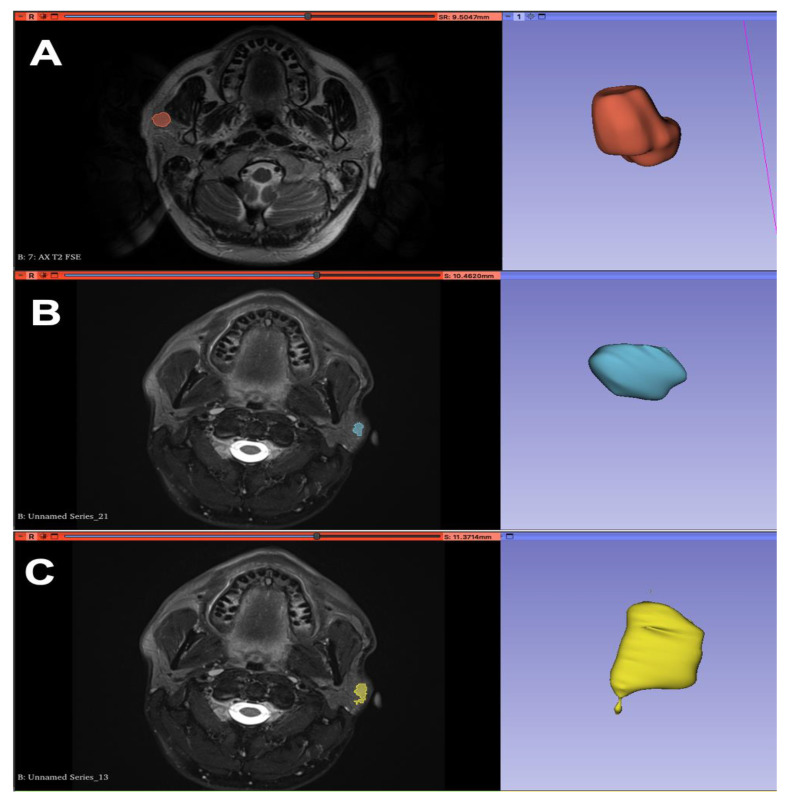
Segmentation of MRI images in axial T2 projection with 3D reconstruction of the ROI (region of interest) using 3D Slicer software: pleomorphic adenoma (A), Warthin tumor (B), malignant neoplasm (C).
